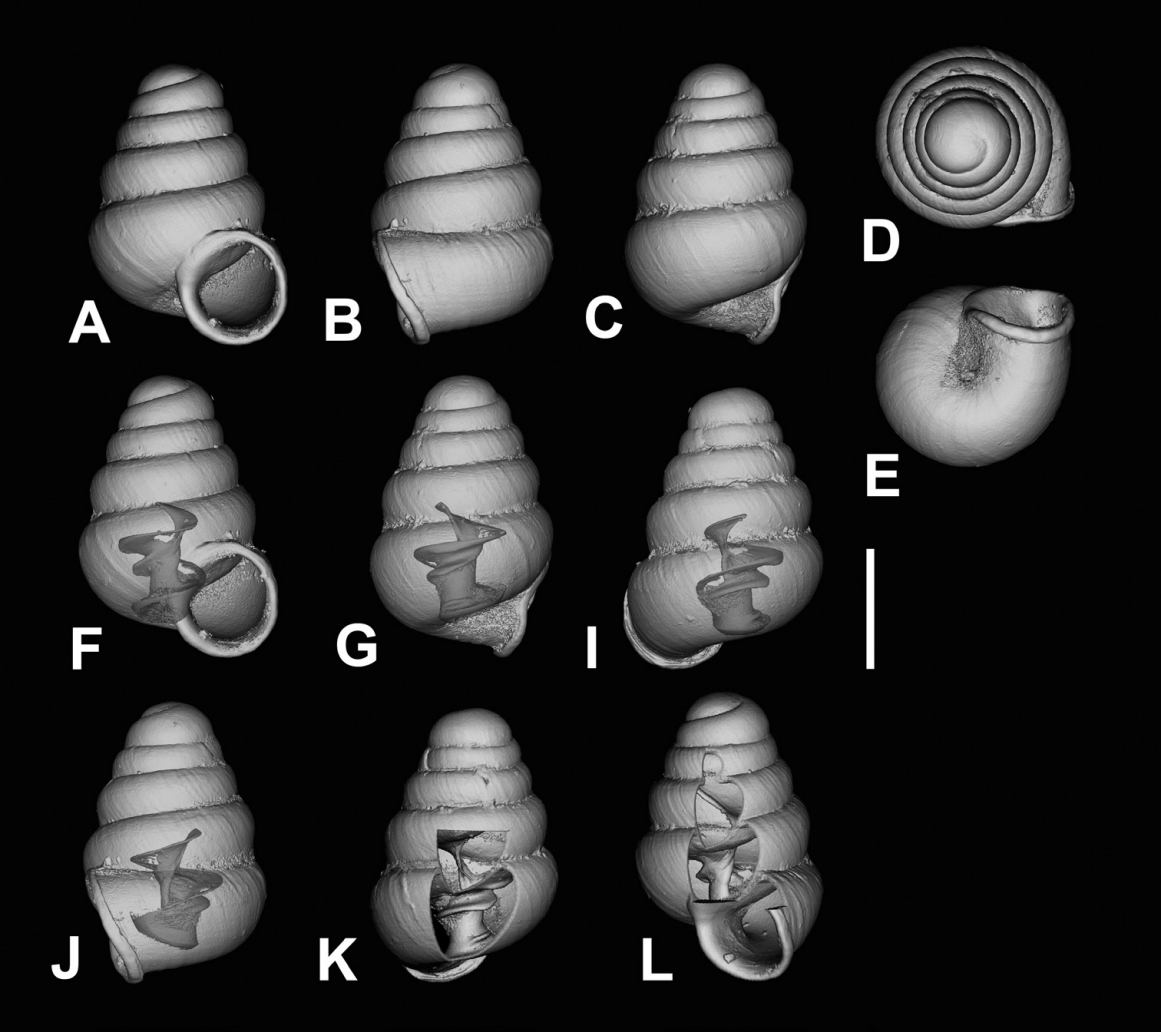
CT images of *Zospeumpraetermissum* sp. n. holotype (RMNH.MOL.55391). F inclinate lamella G, I, K show upper lamellar bulge, central lamella, and columellar basal ridge. Scale bar: 500 µm.
